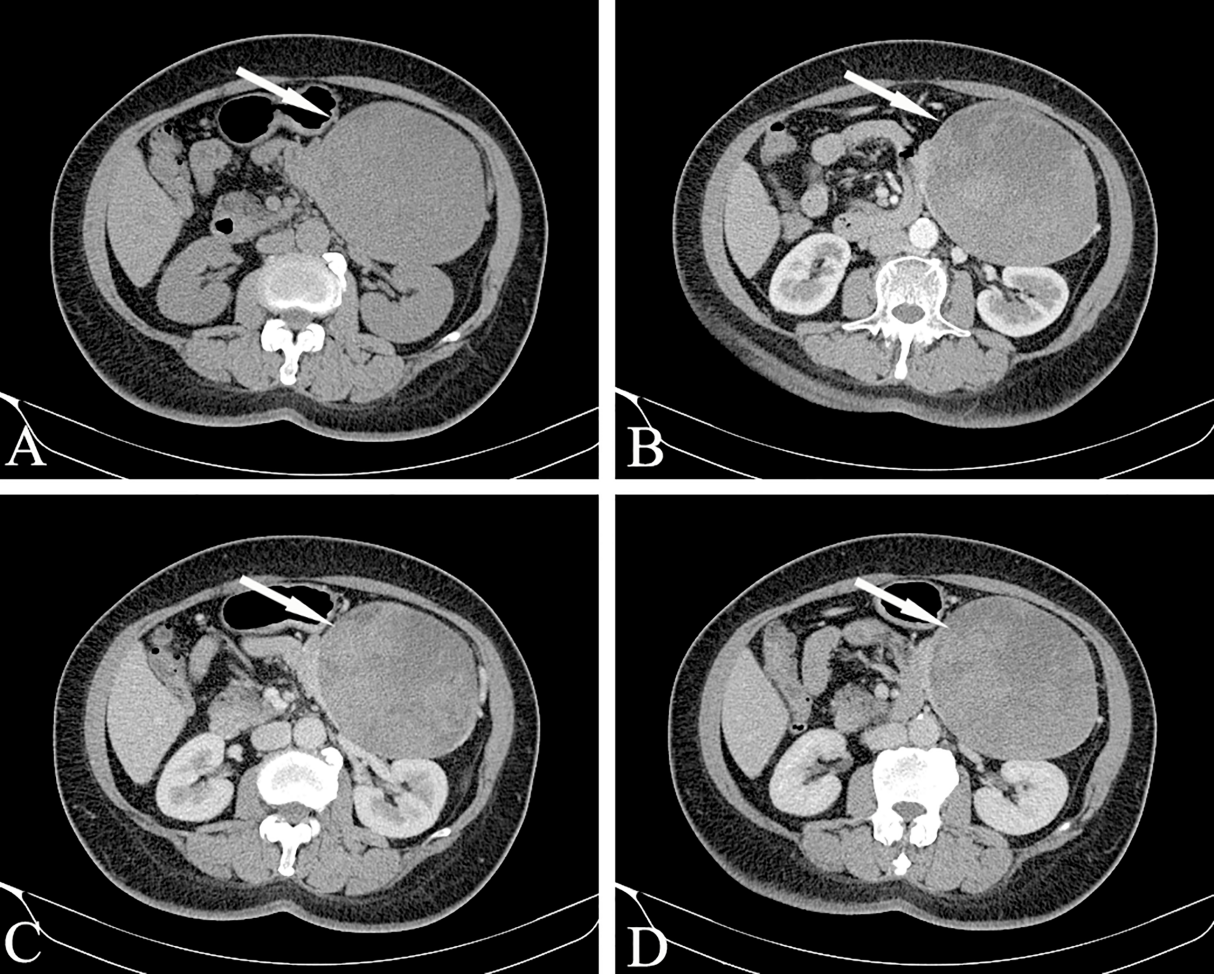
Solid pseudopapillary neoplasm, SPN (Female, 58y, physical examination revealed a pancreatic mass for one month). (A–D) The CT plain scan, arterial phase, portal phase and venous phase at the same level. The lesions are large in size, with cystic and solid components visible inside. The cystic part is not enhanced, but the internal solid components are enhanced and distributed in patches in low-density liquid tissue, showing the “floating cloud sign”. Besides, note that the pancreatic tissue had a “cup-mouth” boundary. The white arrows in the Figures only indicate the location of the neoplasm.
